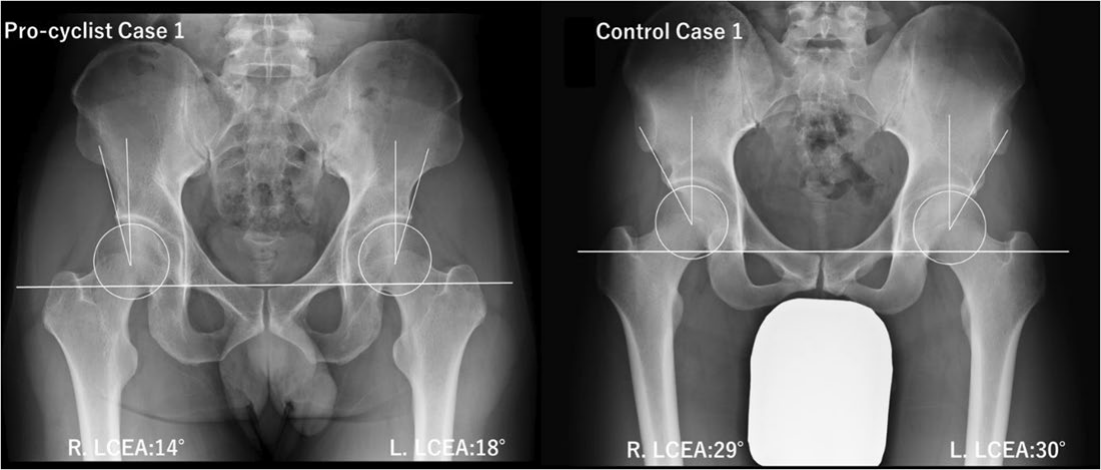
The anteroposterior radiographs and corresponding LCEA data for Pro-cyclist Case 1 and Control Case 1
